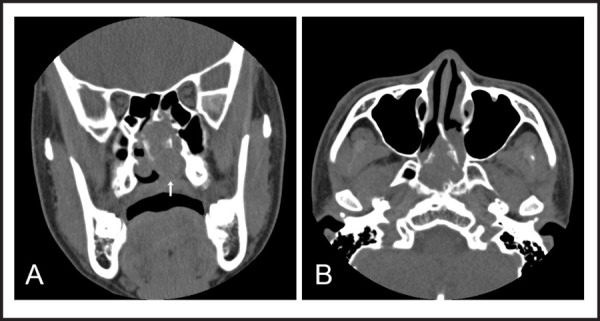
(A) Coronal view of computed tomography scan shows about 4.0 x 3.0 cm heterogeneously enhancing mass which obliterates the nasopharynx and attaches to the basisphenoid and soft palate (white arrow), (B) Axial view of computed tomography scan shows posterior nasal septum which was destructed by the tumor.
